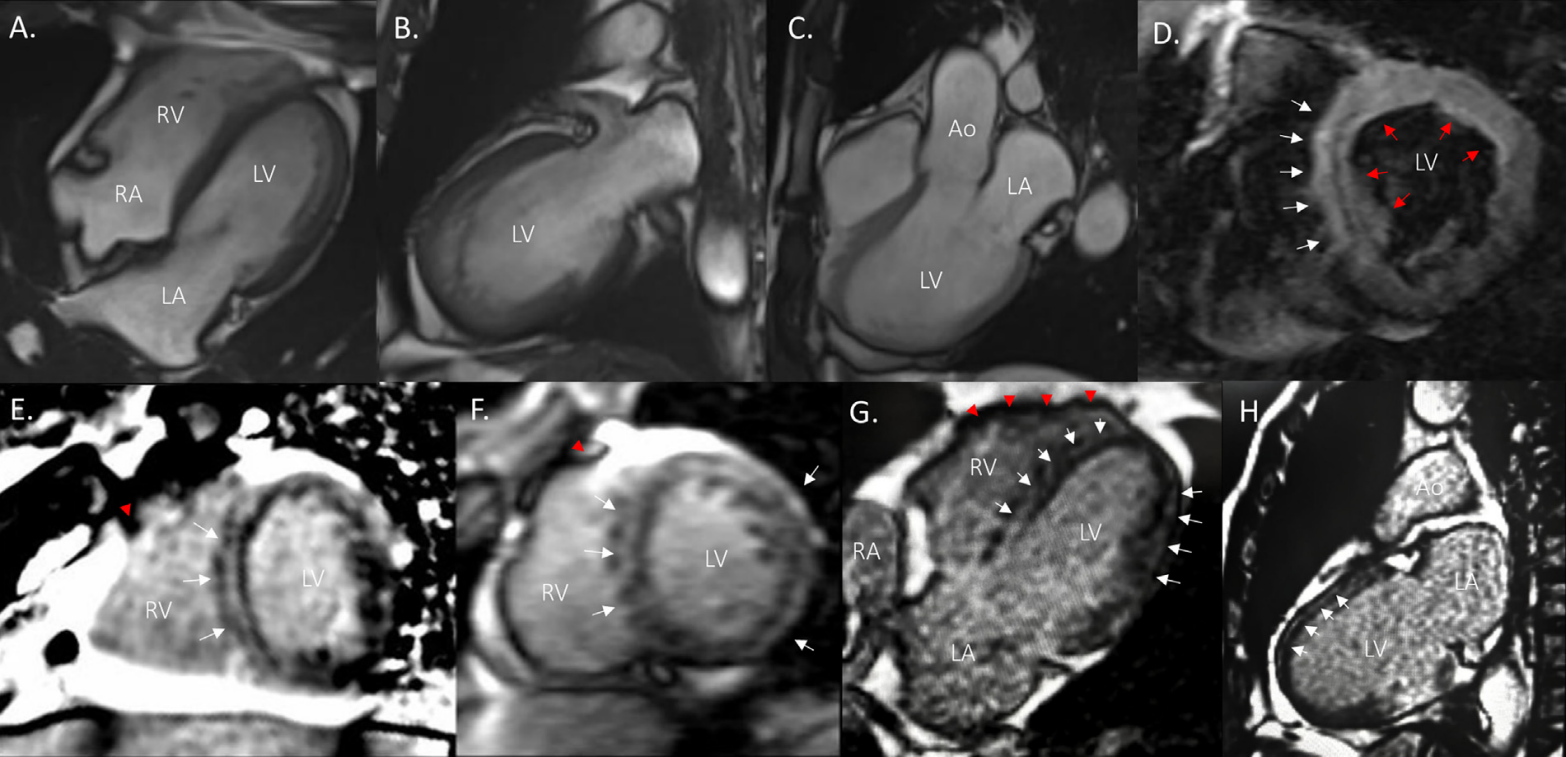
Gadolinium-based magnetic resonance imaging. End-diastolic bSSFP cine (A-C) showing biventricular enlargement. (D) T2-weighted short-axis view showing a linear hypointensity signal in the basal third of the interventricular septum (white arrows) and hyperintensity (red arrows) which likely represents edema. (E, F) LGE short-axis view showing LV ring-pattern subepicardial enhancement (white arrows) and irregular morphology of the RV free wall (red arrowheads). (G) Four-chamber view demonstrating diffuse LGE involving the subepicardial layer of the LV lateral wall and the interventricular septum (white arrows) and showing irregular morphology of the RV free wall (red arrowheads). (H) Vertical long-axis view showing subepicardial LGE at anterior LV wall (white arrows). Ao, Aorta; LA, Left atrium; LV, Left ventricle; RA, Right atrium; RV, Right ventricle.
